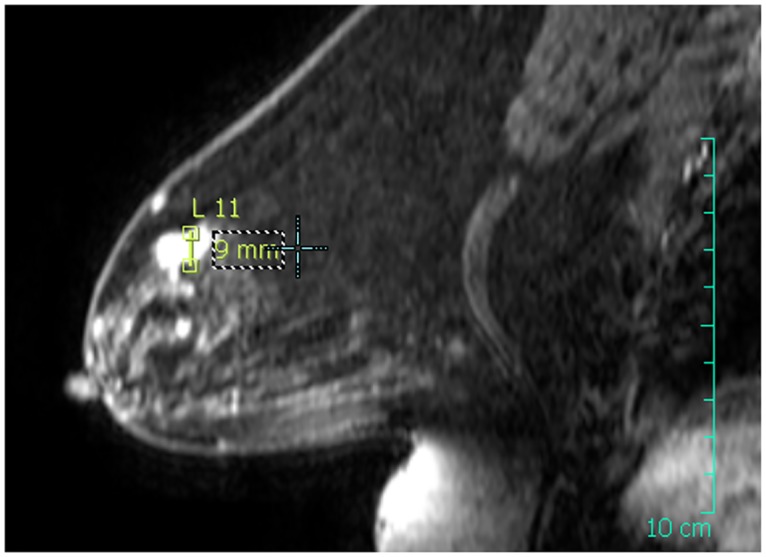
Right breast MRI in the sagittal plane. The dimensions of the tumor were measured and annotated on the MRI image.
